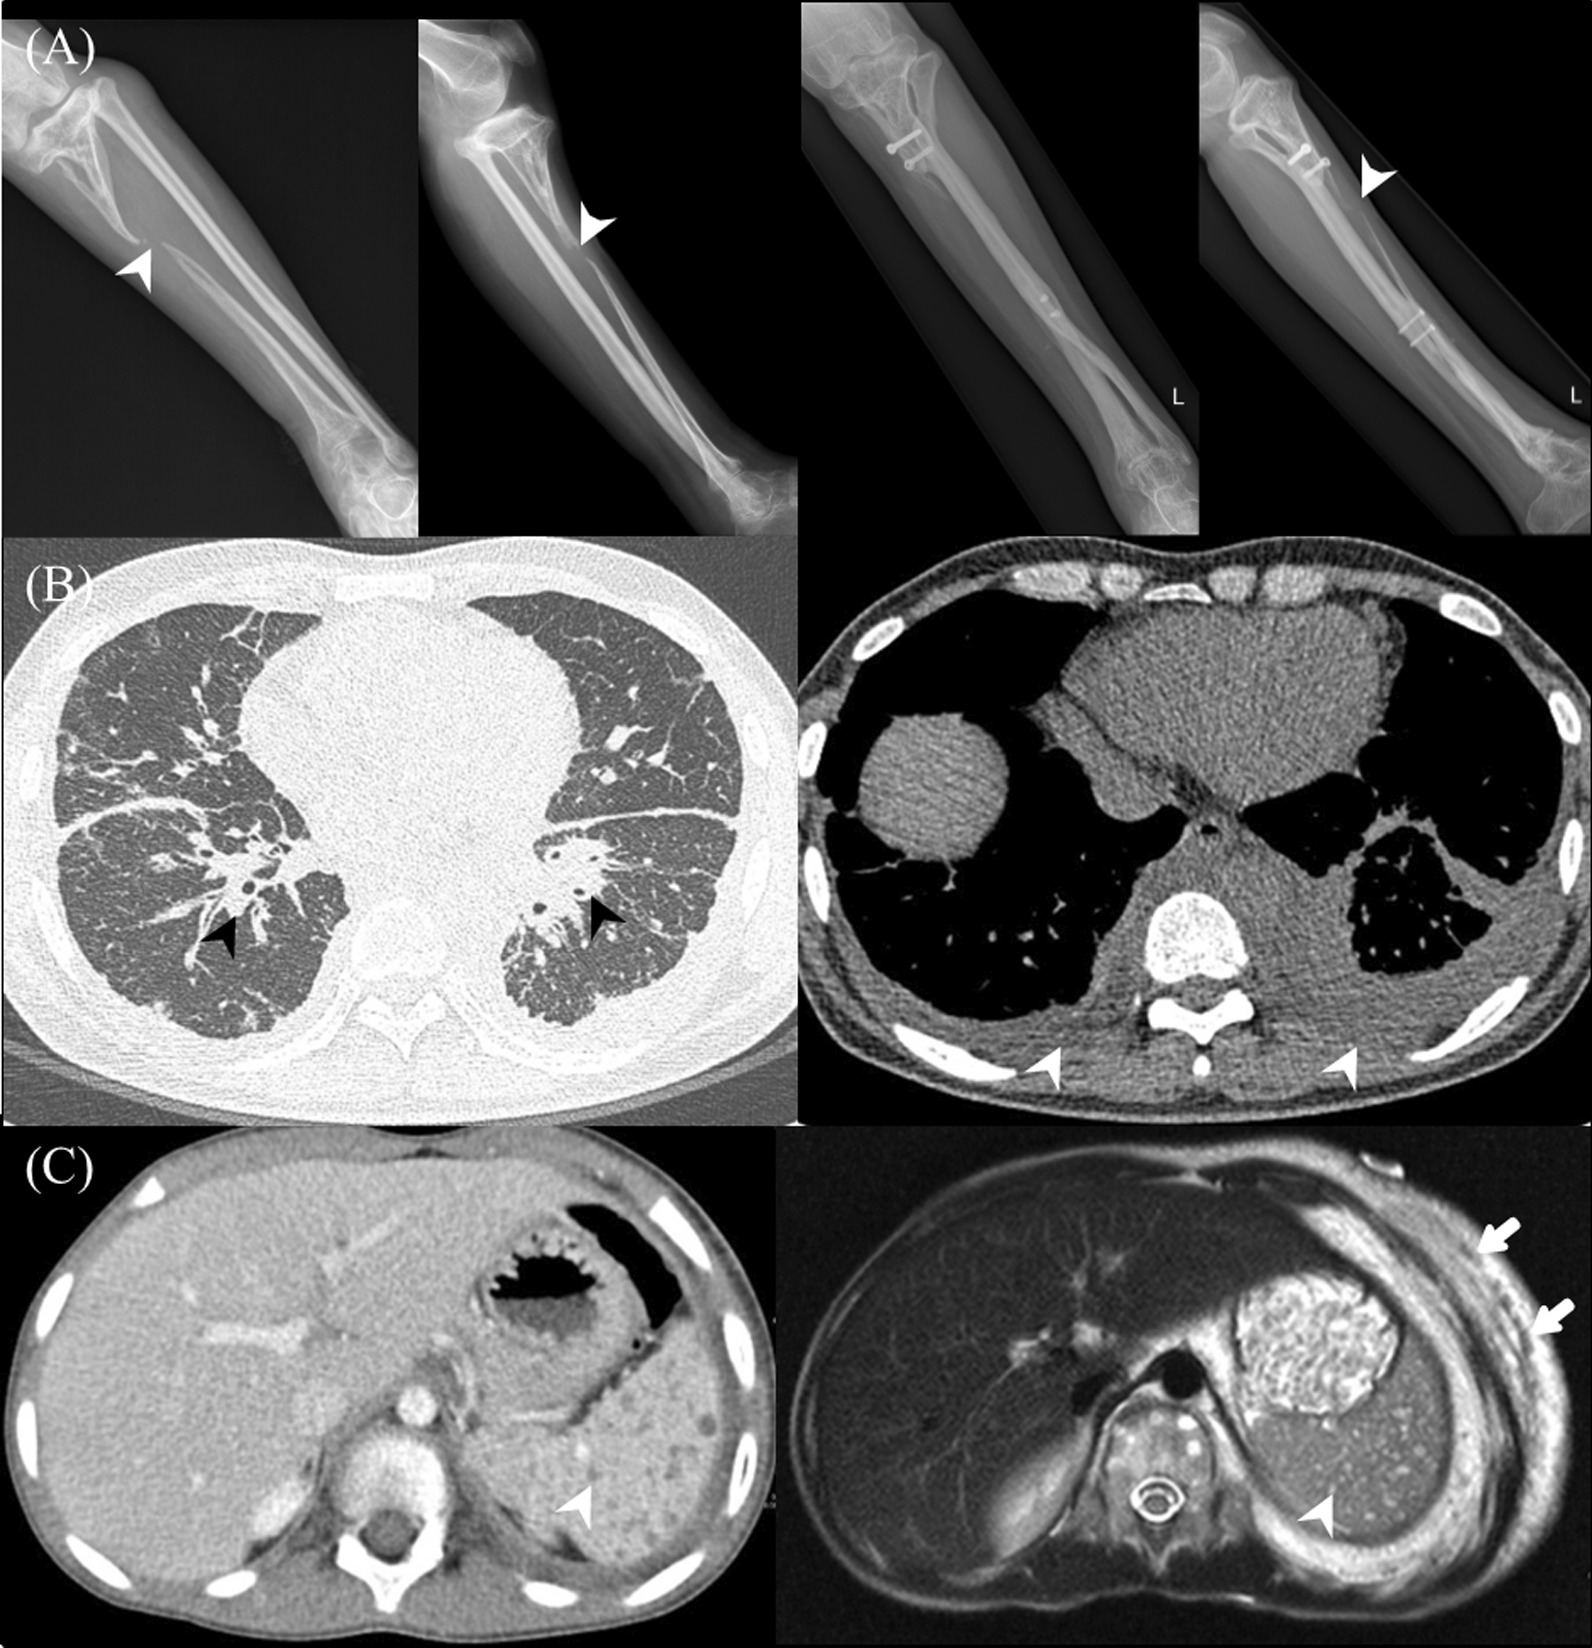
Conventional image characteristics in Gorham–Stout disease. A Plain radiograph images of the left lower leg of a 31-year-old man with Gorham–Stout disease (GSD). A vanishing appearance of left tibial proximal metaphysis and diaphysis (arrowhead) with compensatory hypertrophy of the left fibula is seen (first and second columns). A 7-year follow-up plain radiograph of the left lower leg after surgical correction of deformity showing further progression of the osteolysis (arrowhead) despite the operation (third and fourth columns). B Chest computed tomography (CT) images of a 48-year-old man diagnosed with GSD. On axial non-contrast chest CT images, interlobular septal line thickening and bronchovascular bundle thickening (black arrowheads) were observed in both lungs, with diffuse pleural thickening (white arrowheads) of the bilateral hemithorax. C CT and magnetic resonance images of a 22-year-old man diagnosed with GSD. On axial contrast-enhanced abdominal CT images (first column), multiple small, low-attenuated nodular lesions (arrowhead) were observed in the spleen, but there was no splenomegaly. On axial T2-weighted images (second column), these lesions showed high T2 signal intensities (arrowhead). These could be considered as the splenic manifestation of GSD. Further, there were prominent soft-tissue lesions in the anterior mediastinum and collaterals along the left chest wall (arrows)
